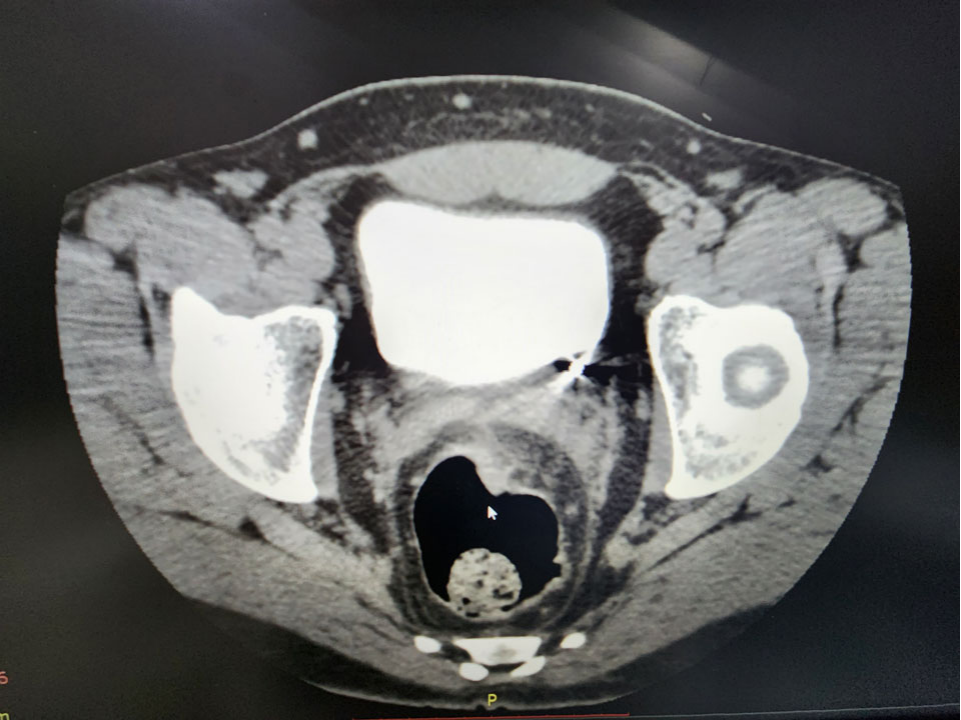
CT of the lower abdomen and pelvis shows a well‐defined metal fragment in the left ureter close to the urinary bladder.
